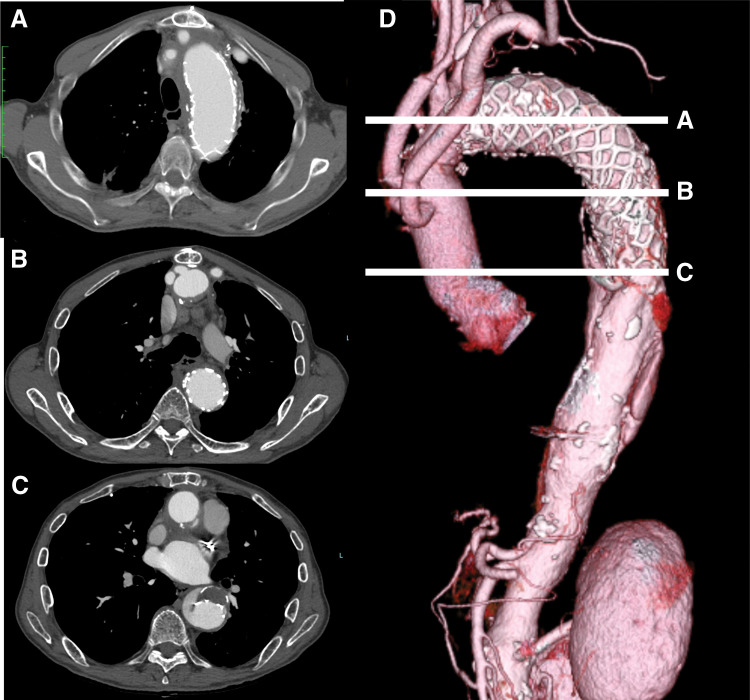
Postoperative CT demonstrating aneurysm exclusion and aortic remodeling. CT axial images at the levels of the aortic arch and proximal descending aorta, bronchial bifurcation, and left atrium at 6 months after surgery (panels A, B, and C, respectively). A 3D CT reconstruction at 6 months is shown in panel D. White lines A, B, and C in panel D correspond to the axial images in panels A, B, and C, respectively.
